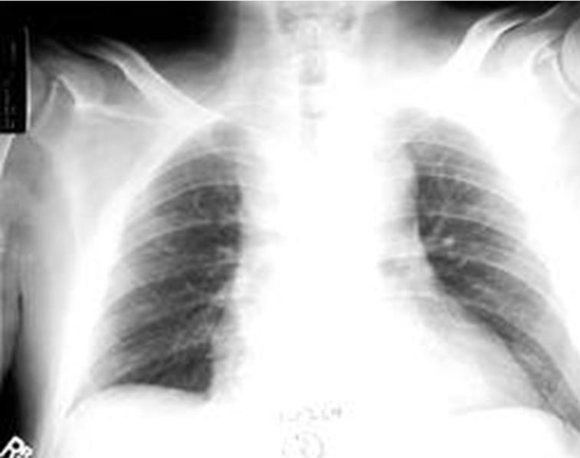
Important élargissement médiastinal (radiographie thoracique de face)
